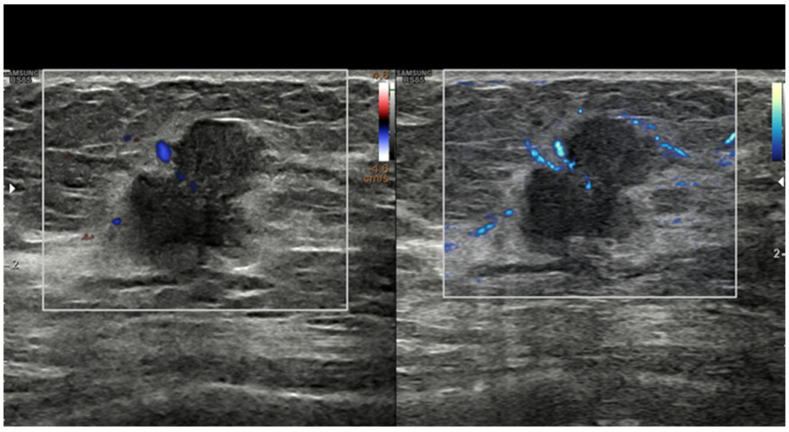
Breast invasive ductal carcinoma. Vascularization as assessed with power Doppler (left) and with MV-Flow (right). Recently developed techniques such as MV-Flow are more sensitive to slow flows and offer a better display of tumor vessels.
